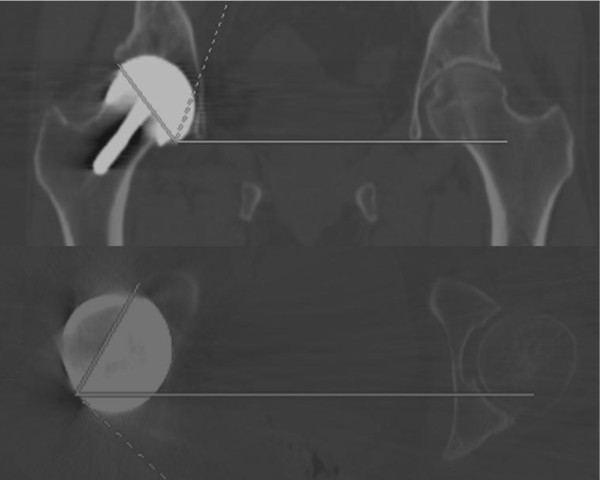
Technique for measuring version and inclination of the acetabular component using CT reconstructions.
